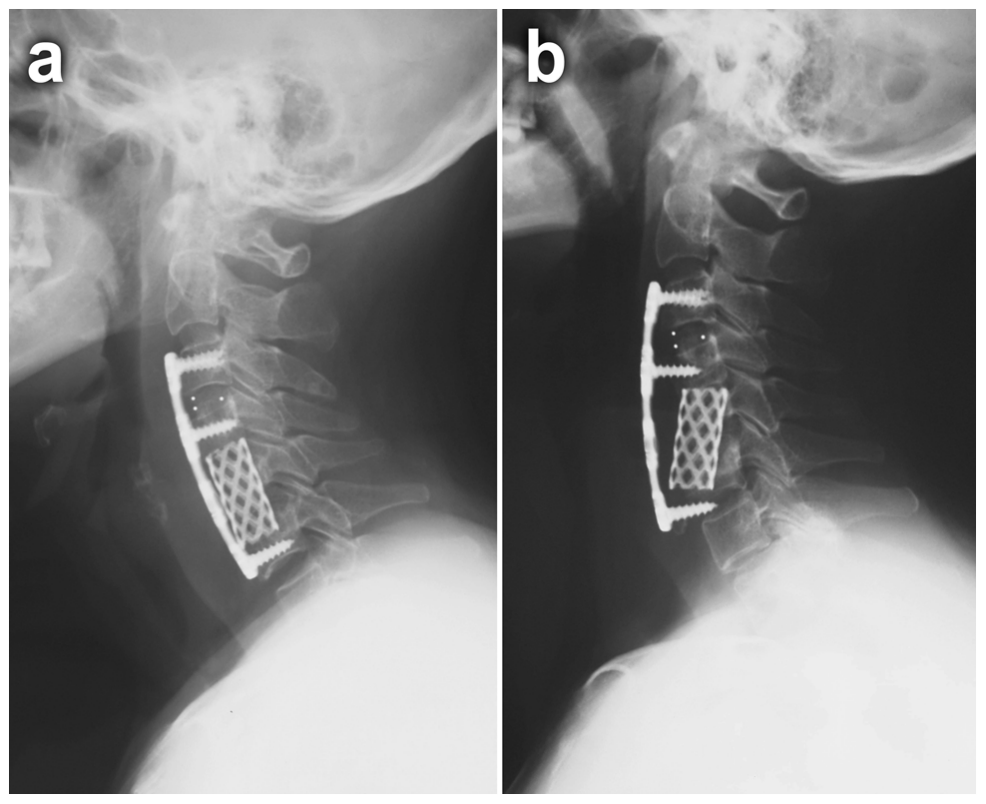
A 62-year-old female patient with cervical spondylotic myelopathy at the C3–C6 level. She was performed with 3-level ACHDF. The postoperative lateral view of this patient showed displacement of the titanium mesh cage and screw loosening at the three months follow-up. a Immediate postoperative lateral X-ray. b 3-month postoperative lateral X-ray.
